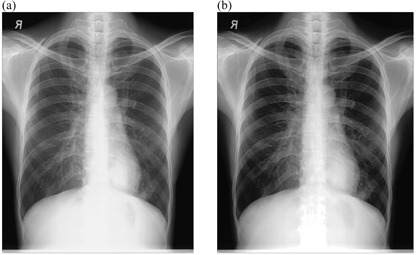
Low kVp chest radiograph: (a) original image and (b) processed image by pixel adjustment and histogram equalization.
